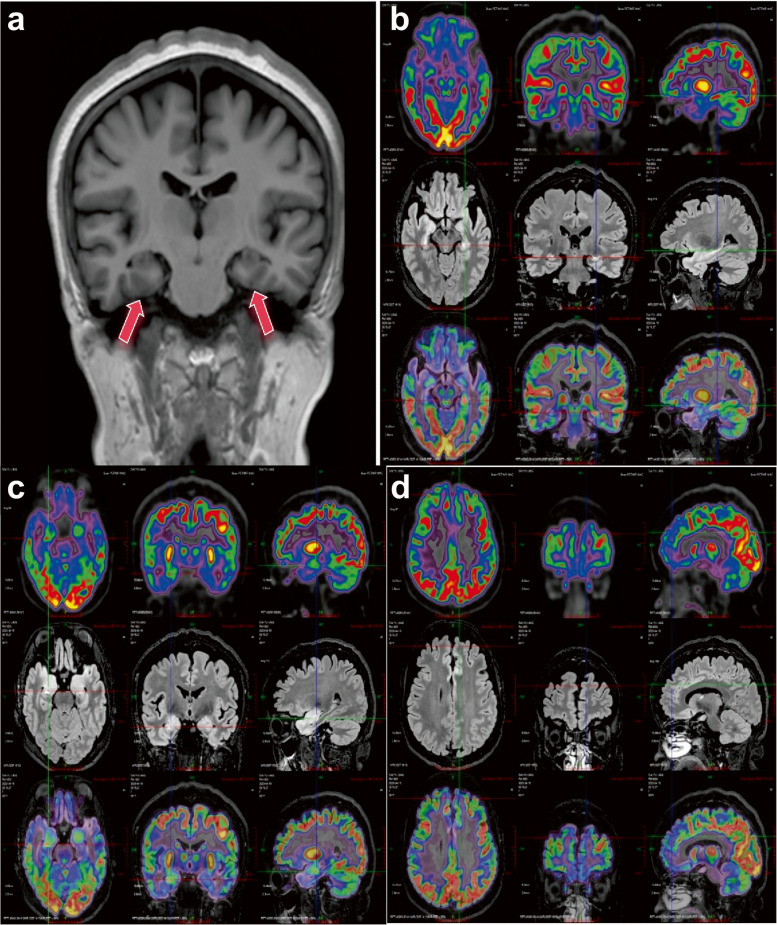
MRI and PET/MR images of Case 7. a MRI showed ill-defined, patchy hyperintensity in the bilateral hippocampi and amygdala on T1-weighted, suggesting encephalitis related changes or other pathology. b,c The bilateral hippocampi and amygdala with abnormal signals showed abnormally increased glucose metabolism, predominant on the left hemisphere (b); d Signal abnormality and symmetrically decreased glucose metabolism in the bilateral anterior medial frontal lobes. The above findings are suggestive of autoimmune encephalitis
